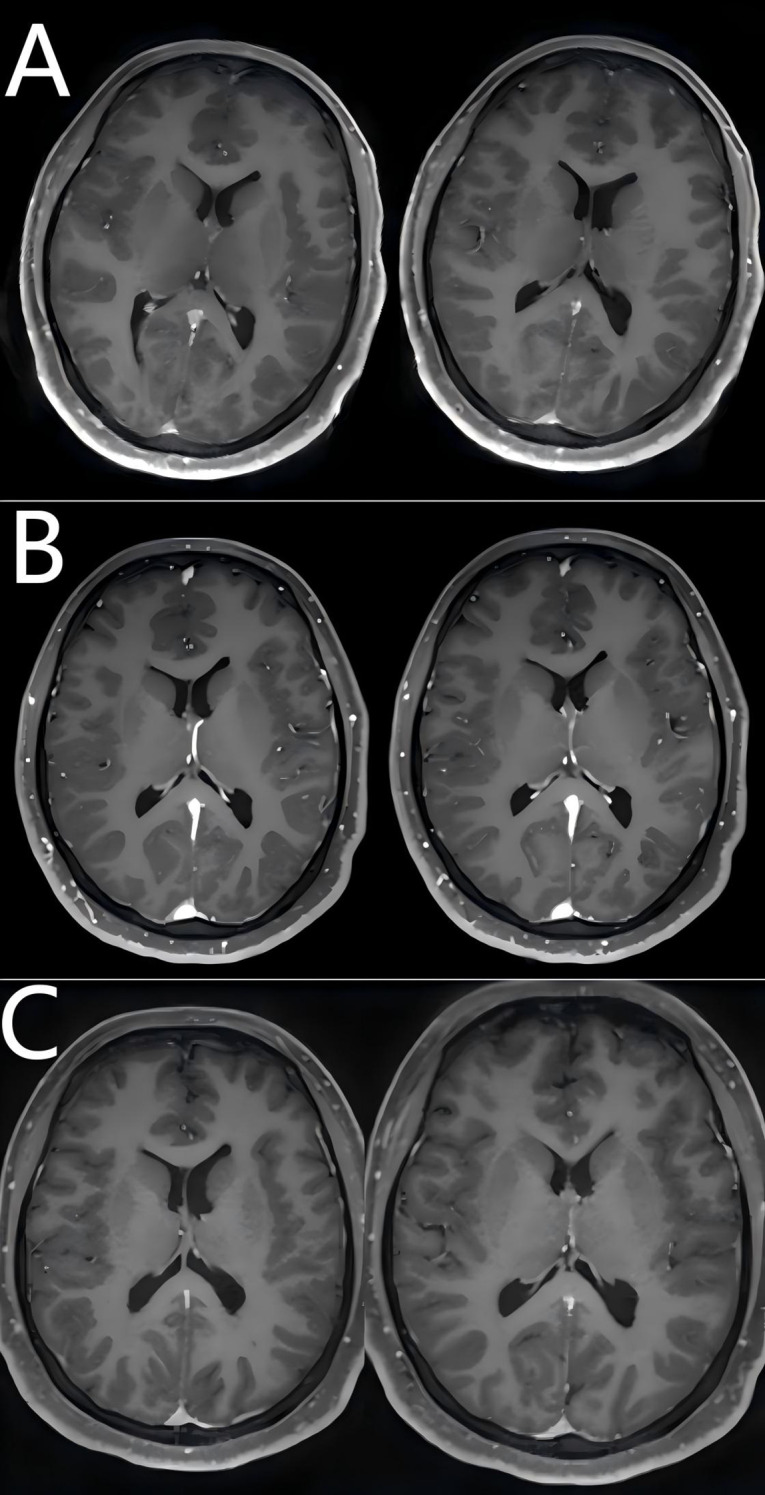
Comparison of pre- and post-treatment brain MRI findings. (A) Brain MRI findings at the diagnosis of viral meningitis (January 1, 2025); (B) Brain MRI findings at the onset of autoimmune encephalitis (January 11, 2025); (C) Brain MRI findings following escalation therapy with first- and second-line agents (March 12, 2025).
